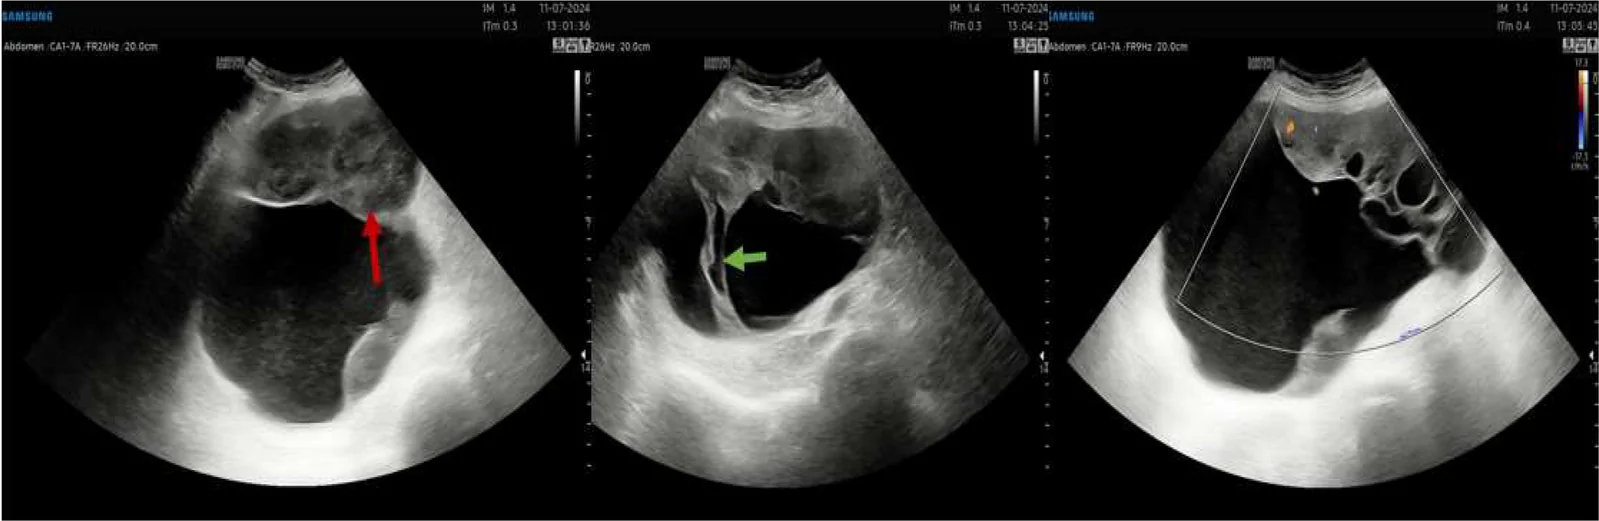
Ultrasound images showing the mass with a fatty component (red arrow), a fluid portion and thickened septa (green arrow).
